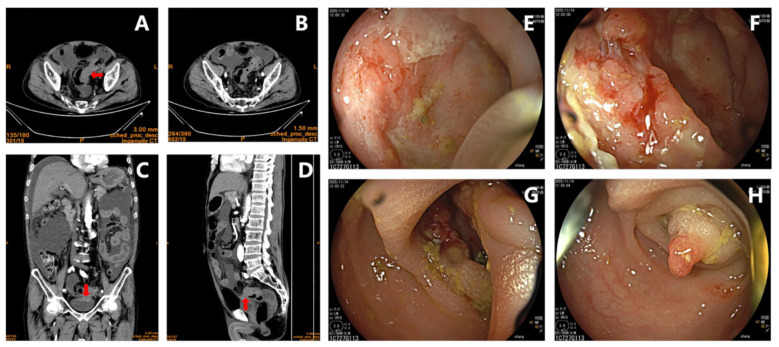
CT and lower gastrointestinal endoscopy findings. (A, B) Axial CT image showing sigmoid colonic wall thickening with multiple pericolic lymph nodes. (C, D) Coronal and sagittal CT images showing thickening and ulcerative disruption of the posterior bladder wall, with an intravesical air-fluid level. (E, F) Endoscopic images showing irregular circumferential ulceration with surface disruption and adherent whitish exudate. (G, H) Endoscopic images at 15 cm from the anal verge showing a circumferential mass-like lesion with luminal stenosis, through which the endoscope could not pass. Red arrows indicate the key CT abnormalities.
